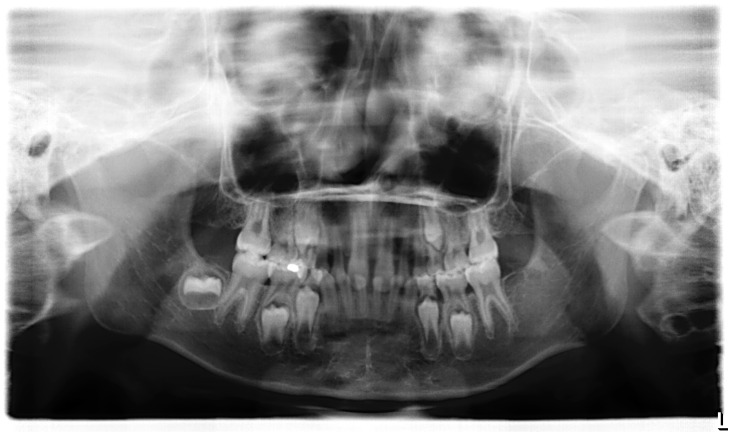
Panoramic radiograph of proband in family 7. He did not have any missing primary teeth congenitally (#51 and #61 had replaced by #11 and #21). However, 15 teeth were missing in the permanent dentition.
